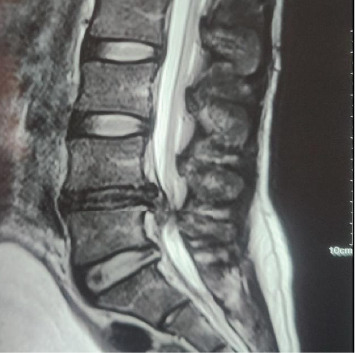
MRI showing broad-based central and right paramedian disc extrusion with caudal migration, causing moderate central canal stenosis at L4-L5 level.
